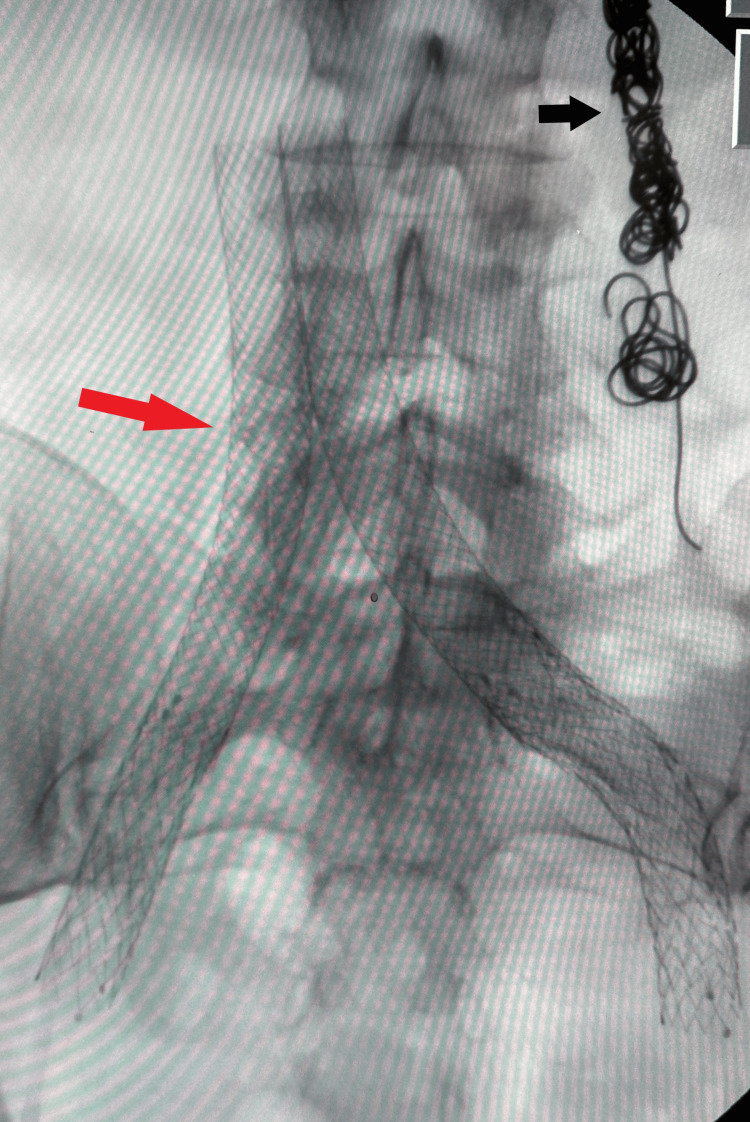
CT image showing stents in the bilateral internal and external iliac veins (red arrow) and coils in the left ovarian vein (black arrow).
